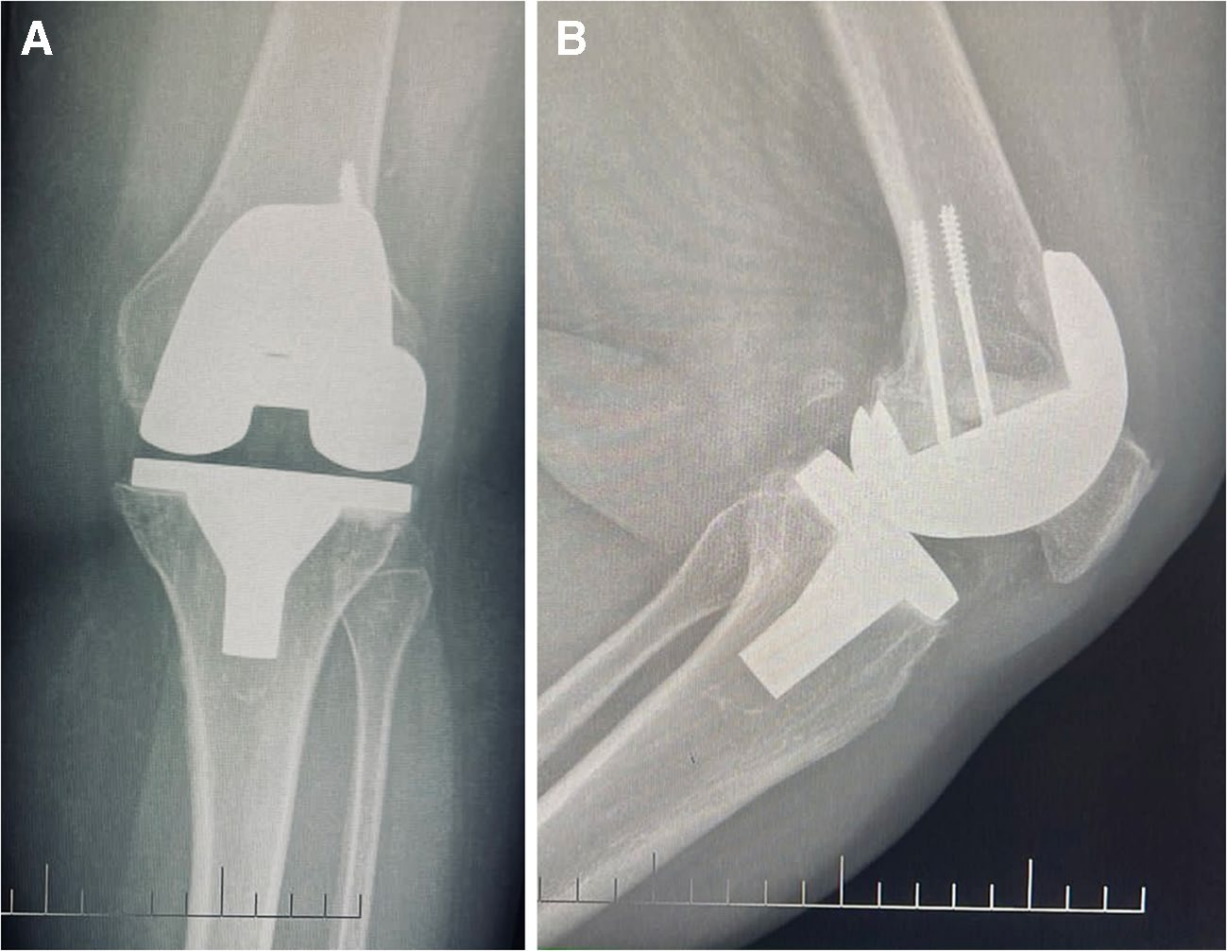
Four years postoperative X-ray of intraoperative lateral femoral condyle fracture fixed by two screws showing healed fracture
